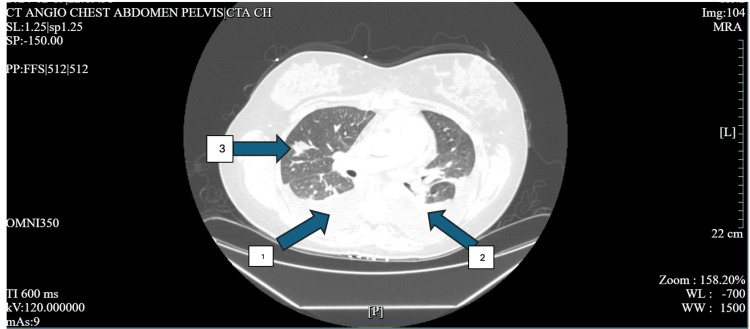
CT angiogram of chest with the right middle lobe infiltrate Arrow 1 demonstrates a right-sided pleural effusion, arrow 2 demonstrates a left-sided pleural effusion, and arrow 3 demonstrates a right middle lobe infiltrate CT: computed tomography
